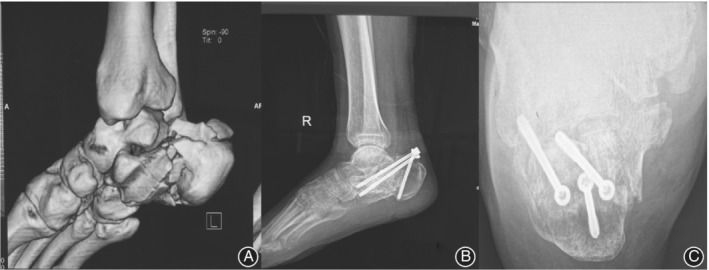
A 45‐year‐old female patient suffered from a Sander type III calcaneal fracture. (A) Preoperative three‐dimensional CT images (B, C). Postoperative X‐ray images showed that the reduction of calcaneal length, height, and width were not ideal.
